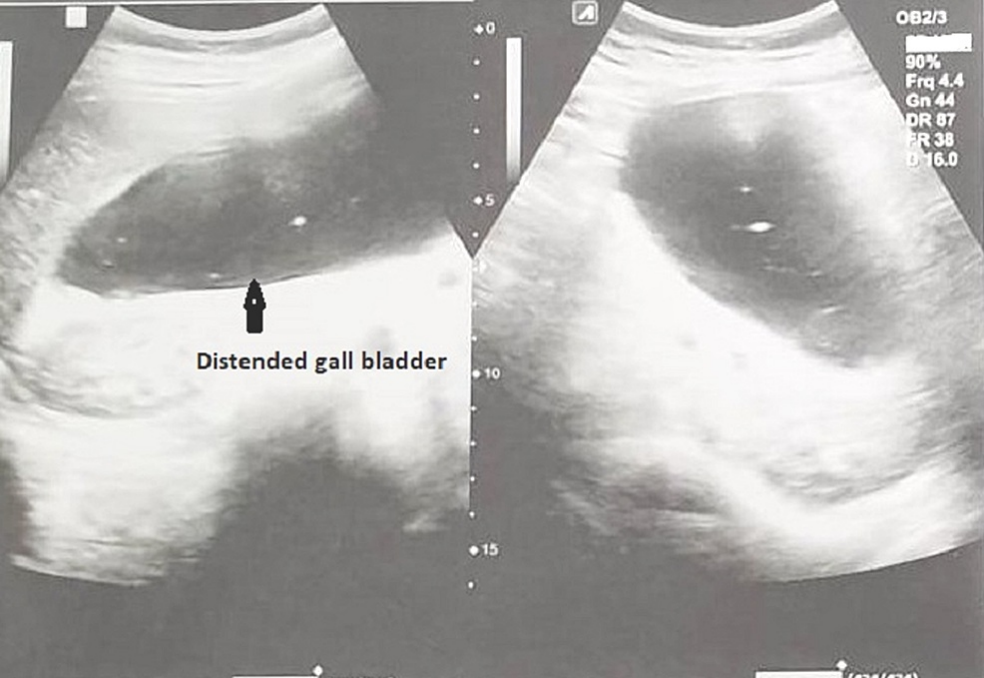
Abdominal ultrasonography showing distended gallbladder
